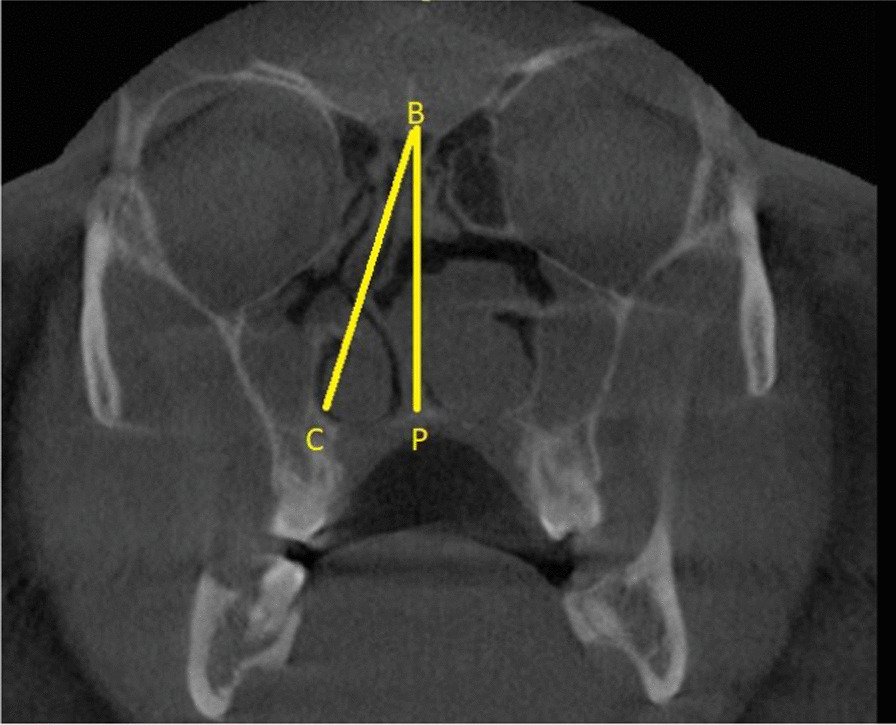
Coronal CBCT section showing the landmarks used for measuring the SDA. The thickening of the sinonasal mucosa was also observed in this image
